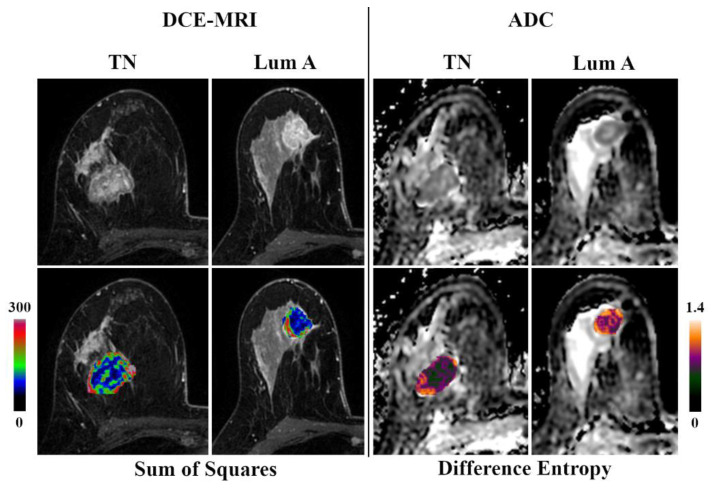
Original DCE-MRI images/ADC maps and corresponding color-coded feature maps as overlays of the tumor area of triple negative (TN) and luminal A breast cancer.
